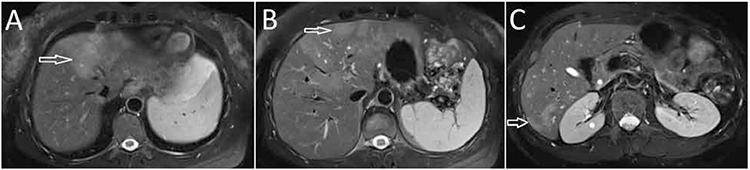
A) Axial T2W magnetic resonance image showing extensive hyper-intense lesions along the portal triads on the left lobe of the liver (arrows), B) A contrast-enhanced T1W magnetic resonance image showing multiple, round, clustered hypo-intense lesions with peripheral contrast enhancement (arrows) in the liver, C) Axial T2W magnetic resonance image showing residual parenchymal hyper-intensity after treatment (arrows)
